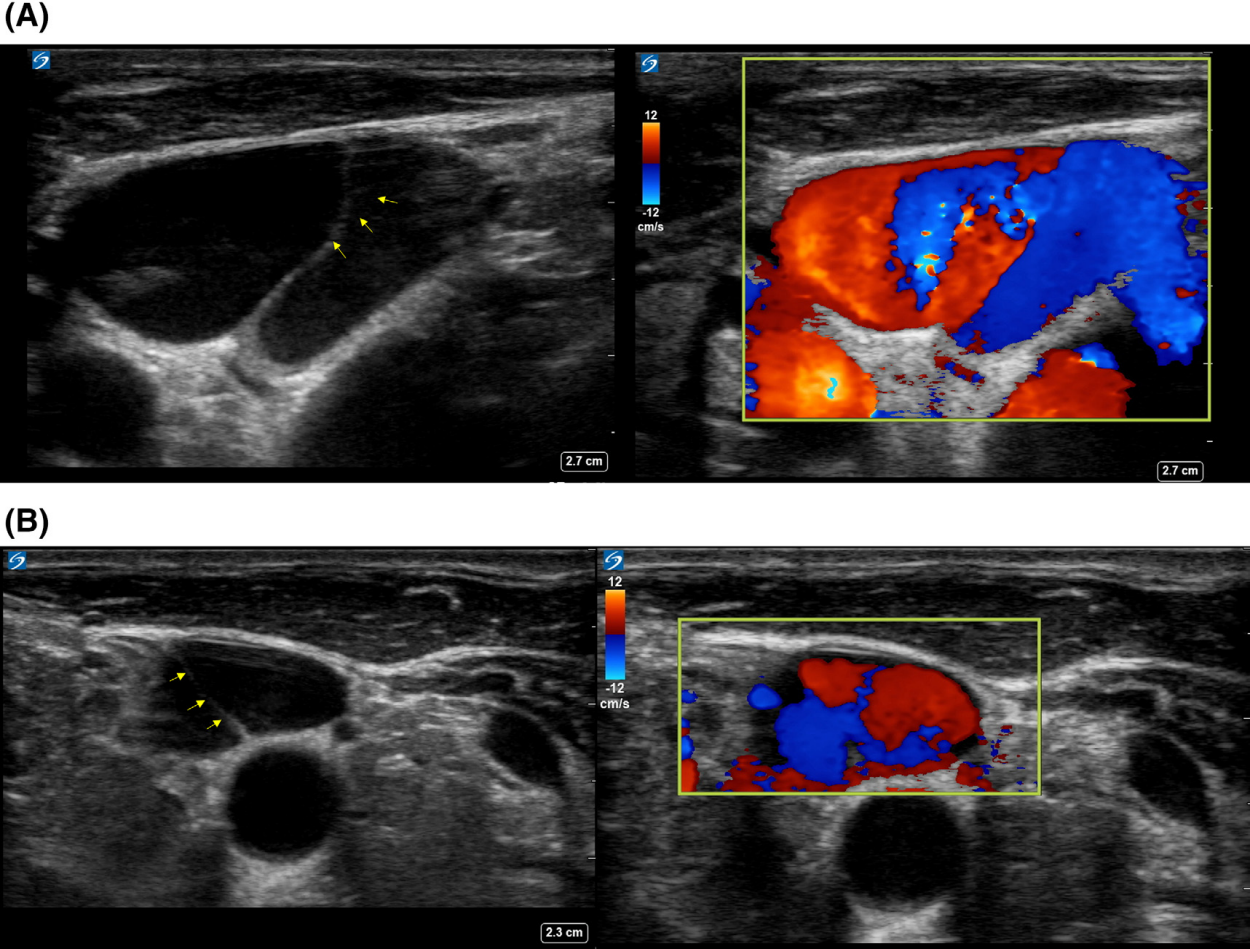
Ultrasound image of (A) right (B) left Internal Jugular Vein. 2D image seen on the left display and Color Doppler evaluation seen on the right display. Arrows point to the mobile structure seen within the lumen of the vein.
